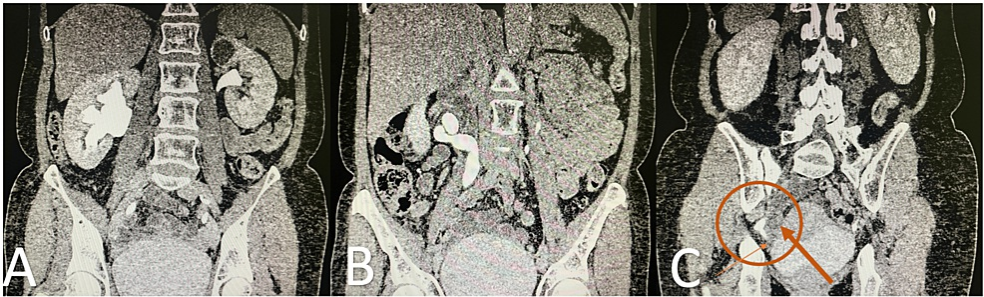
CT scan showing right severe hydronephrosis. A. Sagittal section showing hydronephrosis. B. Sagittal section showing ureteral dilatation. C. Marker: ureter herniating over the sciatic orifice.
